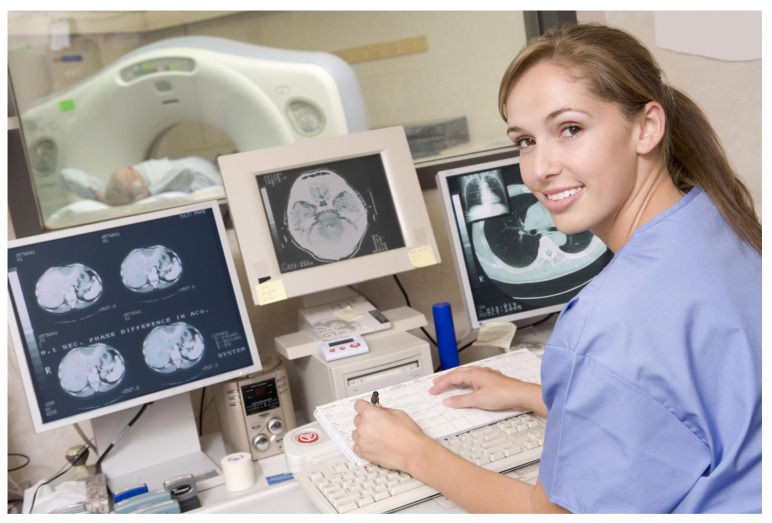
A nurse during a CT examination with the operating software visible (courtesy of @ monkeybusiness/Depositphotos.com). The monitor on the left displays example slices of the abdominal cavity and liver. Liver slices are shown in more detail in Figure 4.
